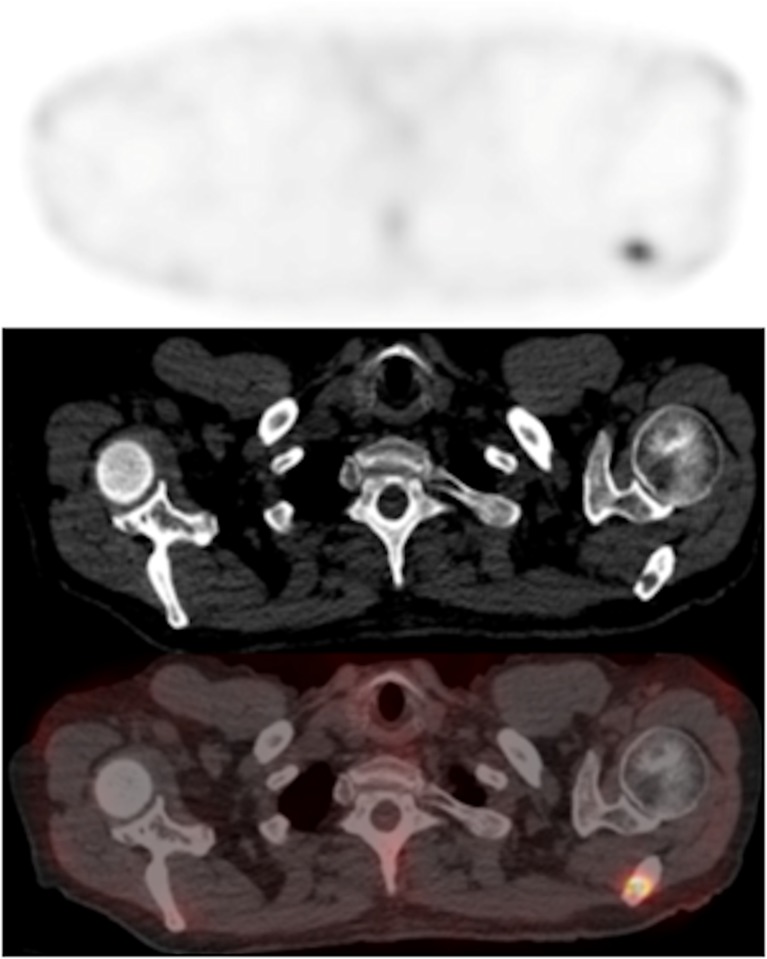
Axial SPECT image (*top*) and axial fused SPECT/CT image (*bottom*) show ^99m^Tc-maraciclatide uptake in a left scapula metastasis (T:N ratio 8.25, T:M ratio 5.91) that appears lytic on the CT image (*centre*; HU = 46)
